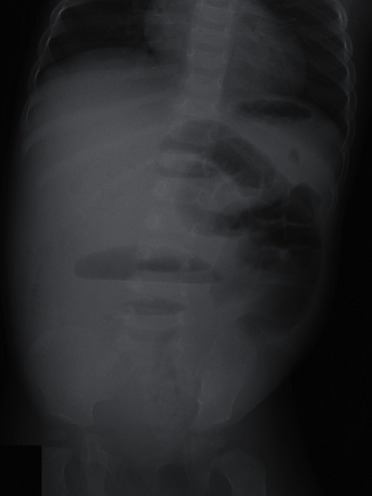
An abdominal X-ray scan demonstrated multifocal air-fluid levels.
